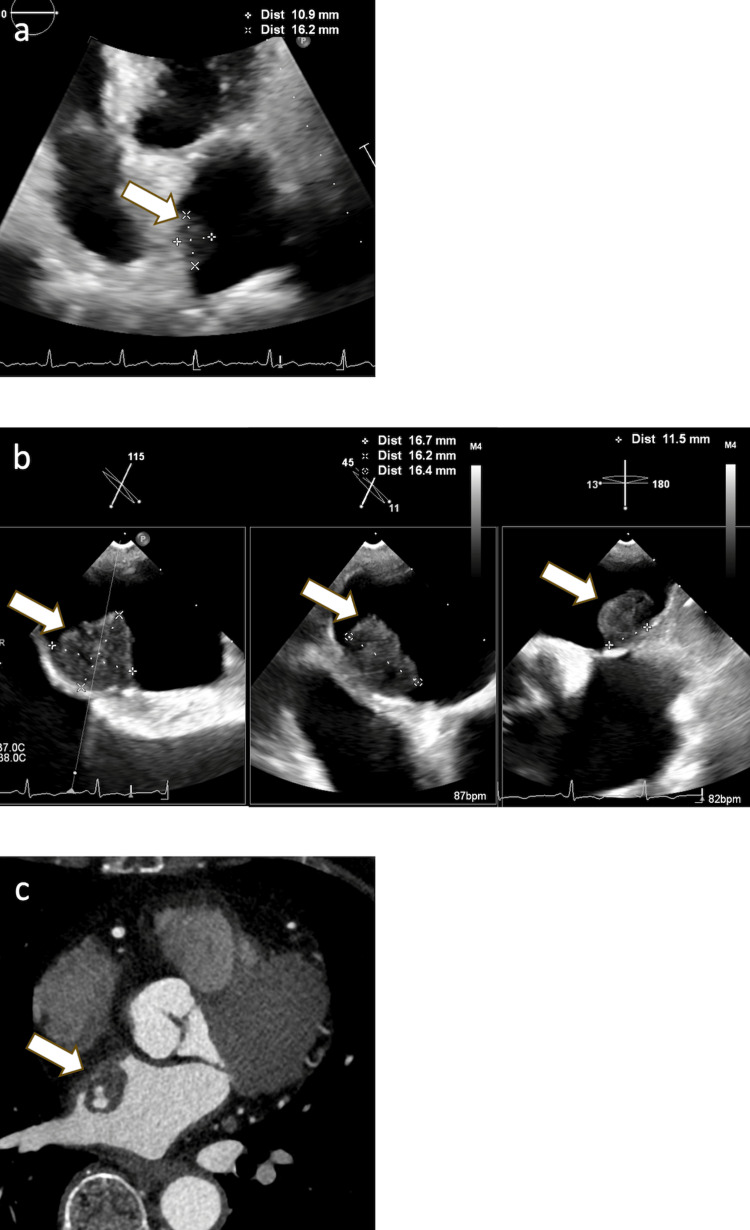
Left atrial mass. (a) Transthoracic echocardiography showed an 11×16 mm mass attached to the left atrial side of the interatrial septum. (b) Transesophageal echocardiography revealed a hypoechoic, irregularly shaped mass measuring 16.7×16.2×16.4 mm with a 10 mm stalk. (c) Contrast-enhanced CT demonstrated a 17×17 mm partially enhancing mass in the left atrium. Arrows indicate the mass in each modality.
